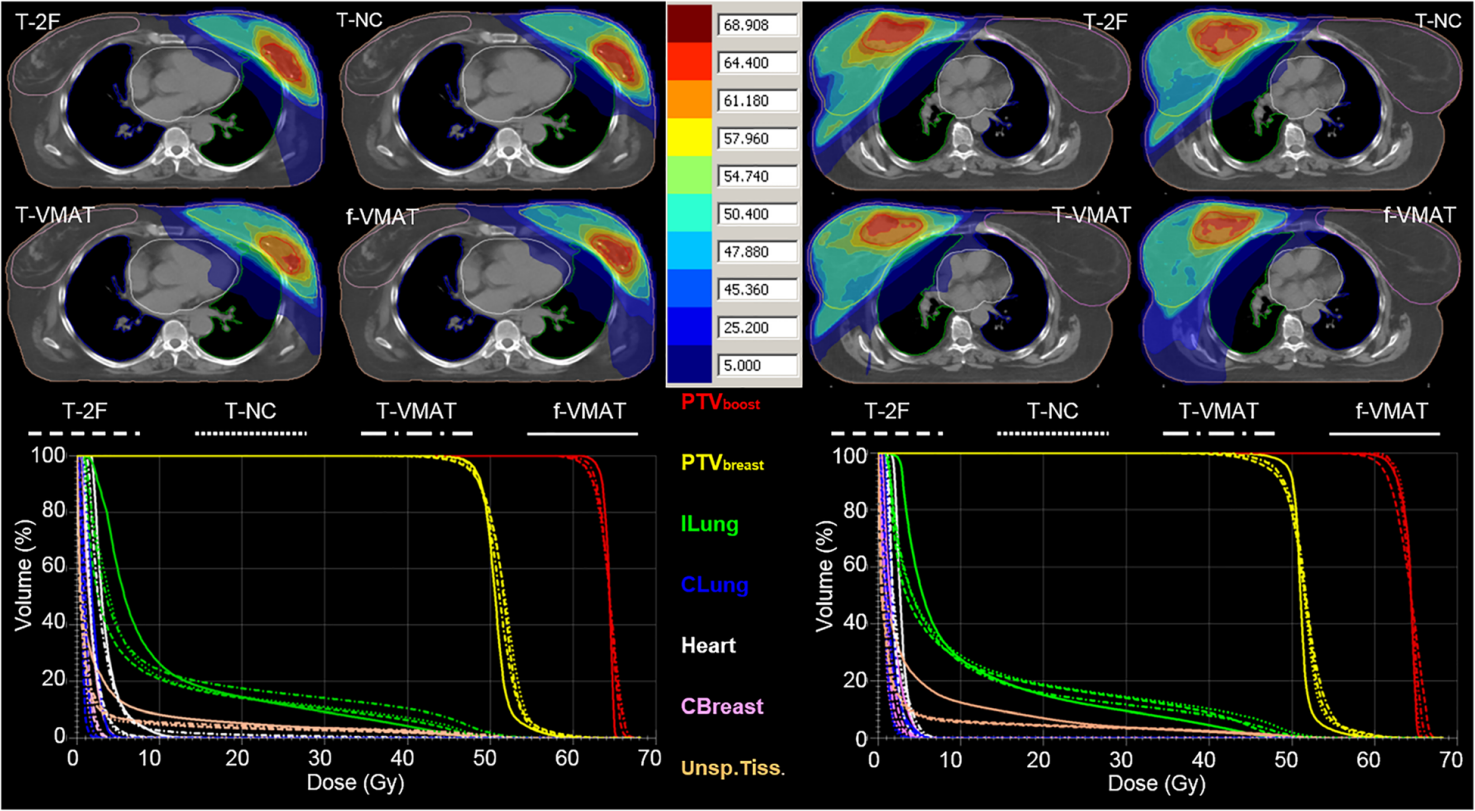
A trans-axial CT slice and the corresponding DVHs of the breast two tangential fields with: two coplanar fields (T-2F), four non-coplanar fields (T-NC), and a VMAT arc (T-VMAT) for the boost volume and a fully modulated VMAT (f-VMAT) techniques for a right-sided (right) and left-sided (left) patient. The DVH line colours correspond to the structure colour
